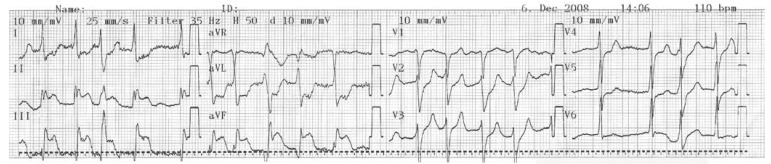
The second CT, 12 hours later, showing extensive infarction of the left frontal area.
